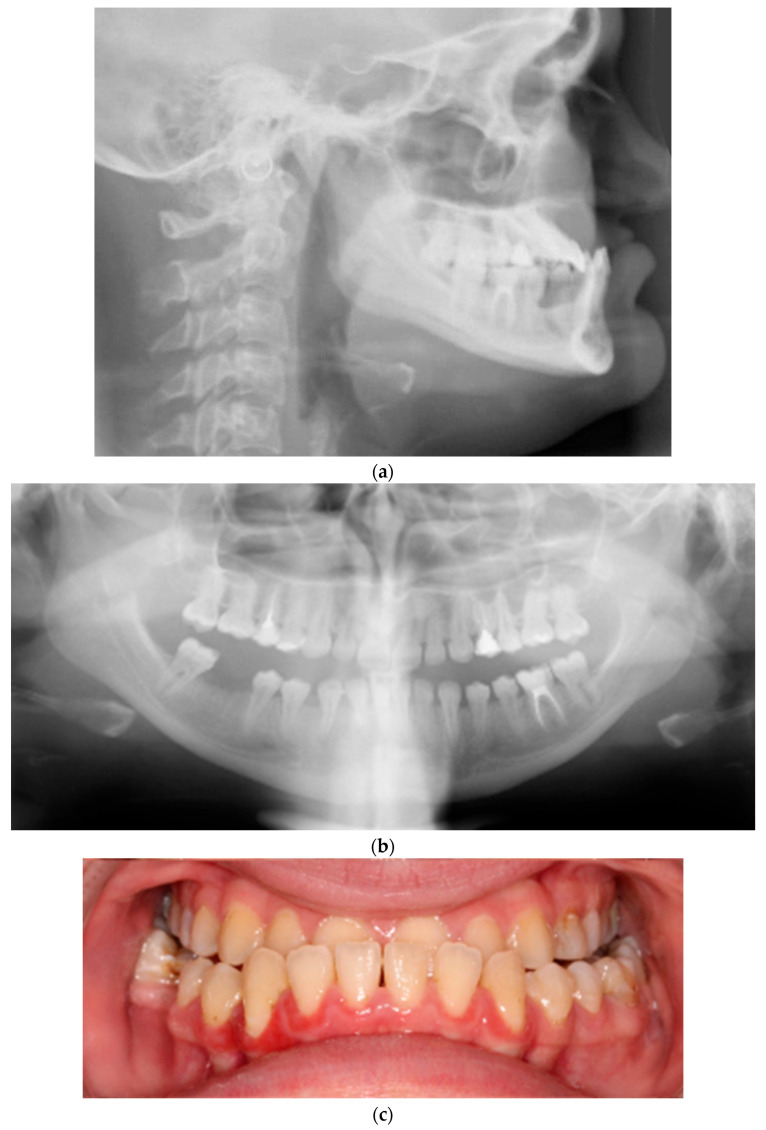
(a) Lateral cephalogram, (b) panoramic radiograph, and (c) frontal intraoral photograph of a 16-year-old female patient with OI. The patient presents a Class III malocclusion, prognathism, and anterior and posterior crossbite.
